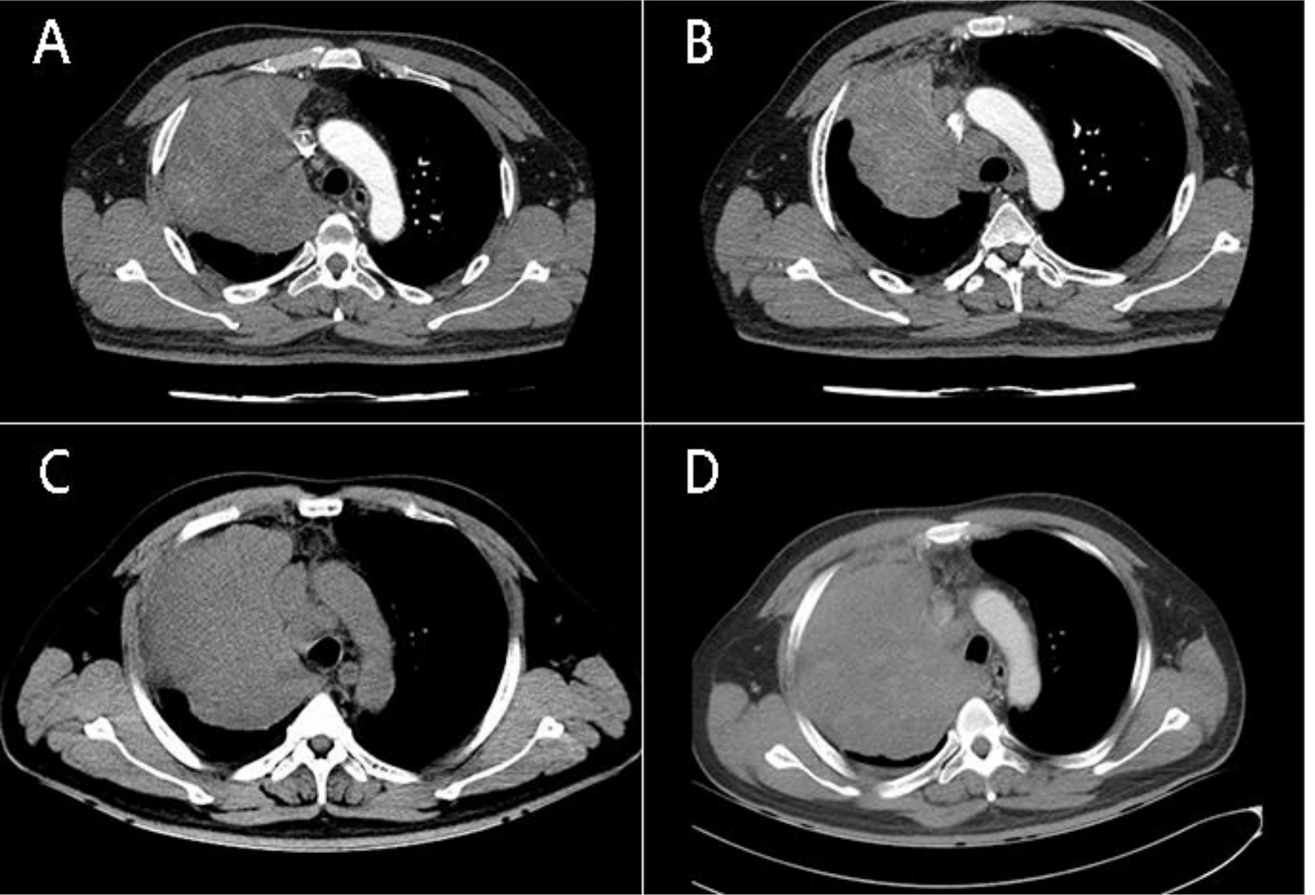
(A) CT of the patient’s chest (July 10, 2018) shows: Massive soft tissue-like density shadows can be seen near the hilar of the right upper lung, the size is about 11.4cm* 10.7cm, the enhanced scan is uneven and moderately enhanced; (B) The patient’s chest CT (September 11, 2018) after VAI regimen chemotherapy showed that the size of the right lung mass was about 8.9*8.1 cm, which was smaller than before, but the mediastinal lymph nodes were enlarged; (C) (September 30,2018) The size of the right lung mass is about 12.1cm*10.5 cm, which is larger than before. (D) (October 11, 2018) The soft tissue-like density shadow of the right upper lobe of the right lung mass, the right hilum is compressed, the main trachea of the right lower lung is compressed and narrowed, and the righthilar and mediastinum have multiple enlarged lymph nodes.
